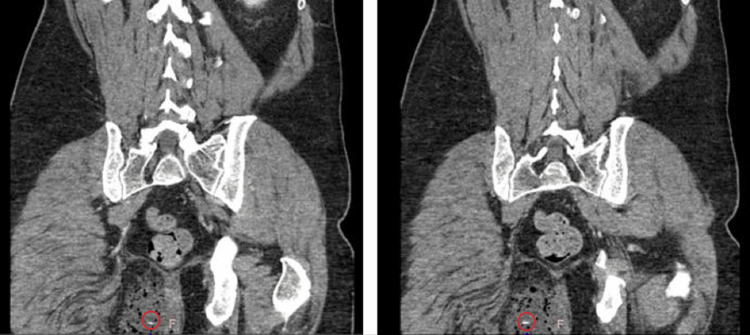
CT scans (consecutive coronal cuts) showing the right gluteal abscess with linear foreign body (red circles)
